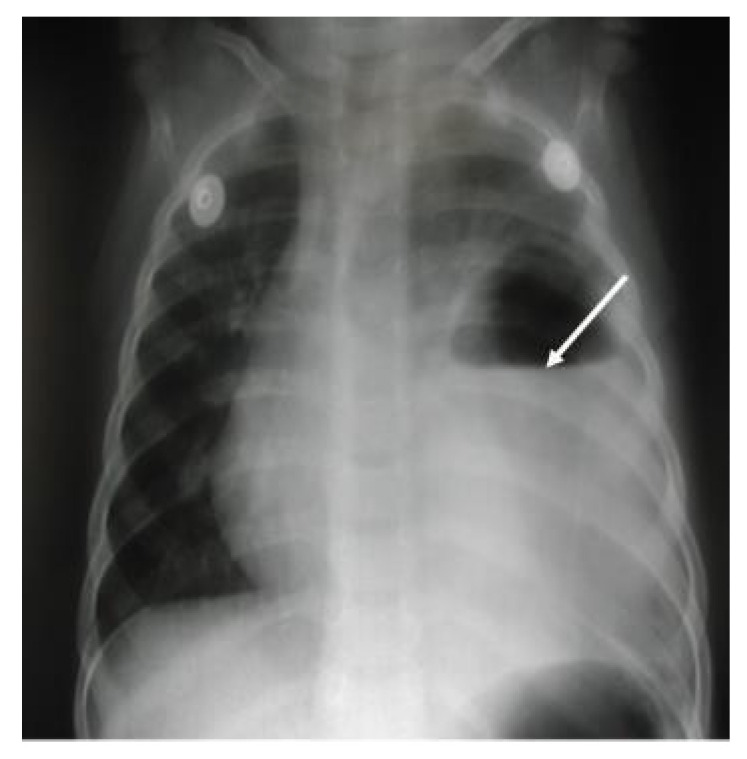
Chest X-ray, AP film, large homogenous density occupying most of the side of the hemithorax with a large amount of air fluid in the superior aspect, which was consistent with a large lung abscess. There was still some shift of the mediastinum to the right, with mild hyperinflation on the right side of the lung.
